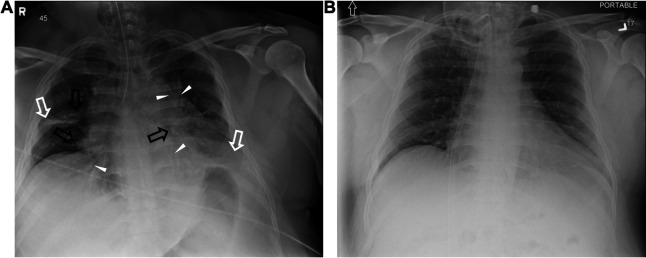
Chest X-rays from admission and post-antimicrobial therapy. (A) AP CXR showing enlarged cardiomediastinal silhouette with low lung volumes, associated right middle lobe, and bilateral infrahilar opacities (black arrows) with air bronchograms (white triangles), fluid in minor fissure, and likely left pleural effusion (white arrows) (day of admission). (B) AP CXR showing improved aeration of bilateral lungs and interval resolution of pulmonary edema and parenchymal opacities, without areas of focal consolidation (day 35 of hospitalization).
